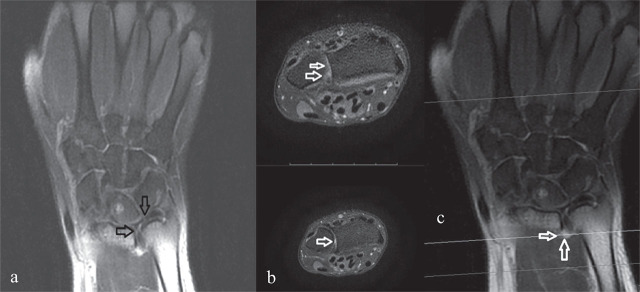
T2-weighted MRI of the left wrist showing: a) increased thickness and signal intensity of the triangular fibrocartilage complex (TFCC) (arrows); b) and c) joint effusion in the distal radioulnar joint (arrows) consistent with a TFCC injury
